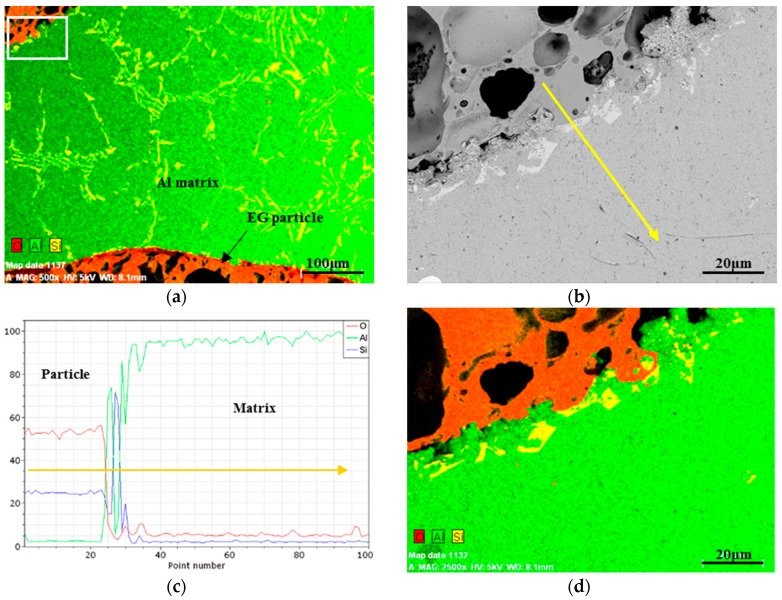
(a) BSE image of base metal; (b) BSE image of the interface; (c) EDS line-scan profiles of the EG-A356 syntactic foam; and (d) elemental analysis image X-ray map of the interface area.
